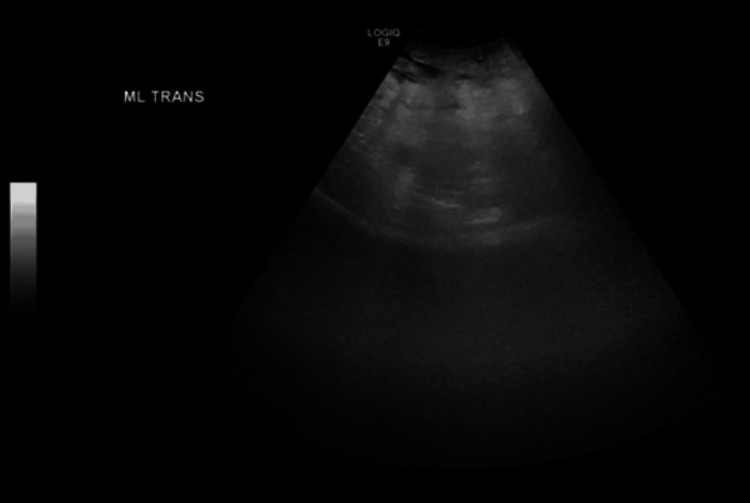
Transverse transabdominal ultrasound image demonstrates a large amount of gas in the lower abdomen limiting the visualization of the pelvic structures due to acoustic shadowing causing a ring-down artifact.
